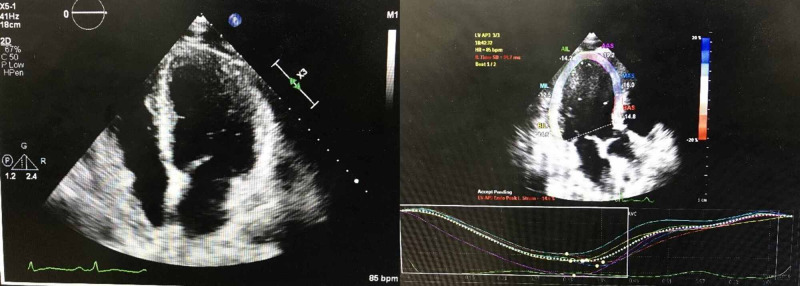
Echocardiography Concentric remodeling with global hypokinesis, grade 2 diastolic dysfunction, and left ventricular ejection fraction of 49.29%
